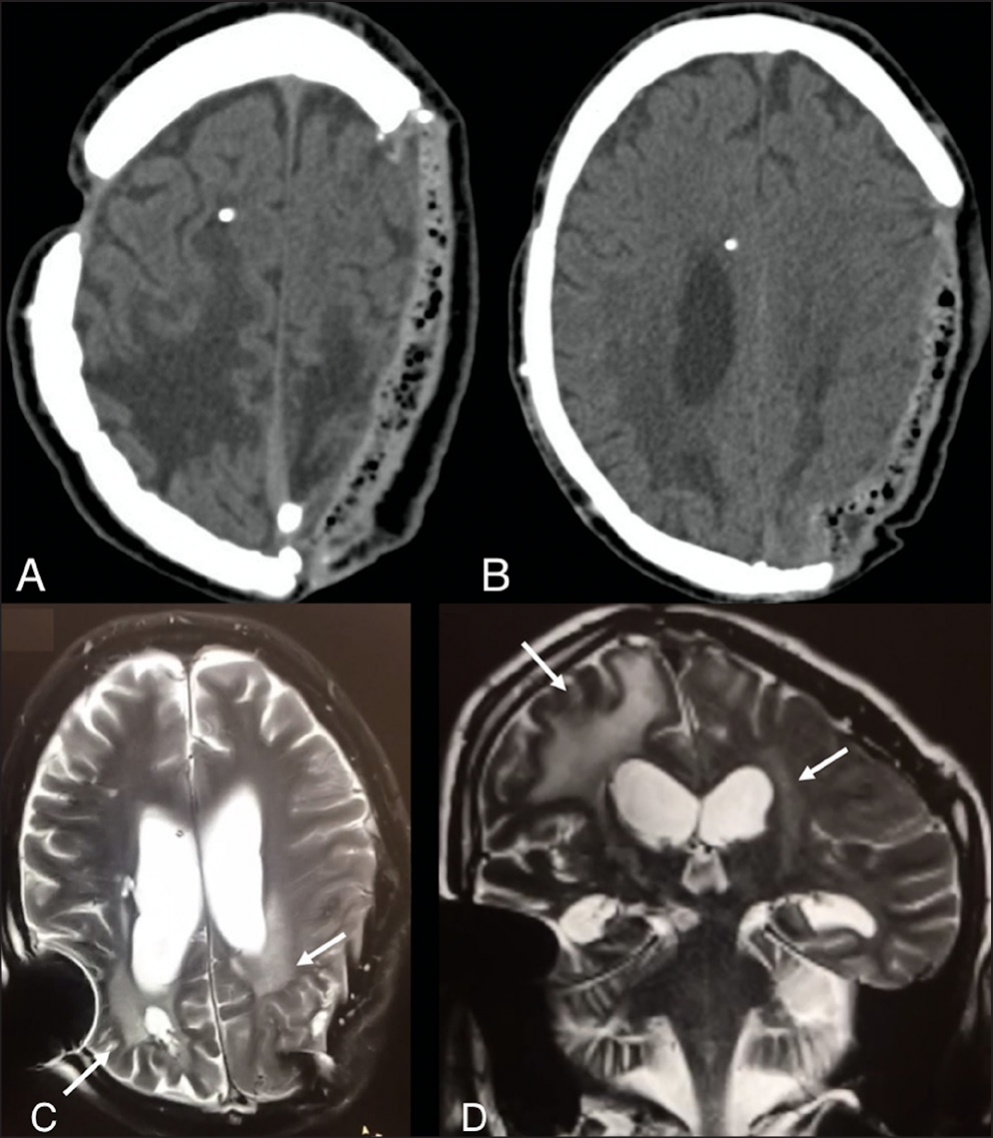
A–B Cranial computed tomography scan showing an extensive left parietal craniotomy, trepanation in the right coronal suture with an external ventricular drain placed in the frontal horn of the right lateral ventricle. Multiple skullcap fractures with misalignment mainly in the right parietal region. High convexity biparietal hypodense lesions with extension to the frontal lobes and adjacent deep white-matter with compensatory lateral ventricles enlargement. C–D: (C) Axial T2-weighted and (D) coronal T2-weighted brain magnetic resonance imaging showing diffuse brain lesions involving the white matter and cortex, particularly in the posterior lobes, related to the previous cranial trauma (white arrows).
